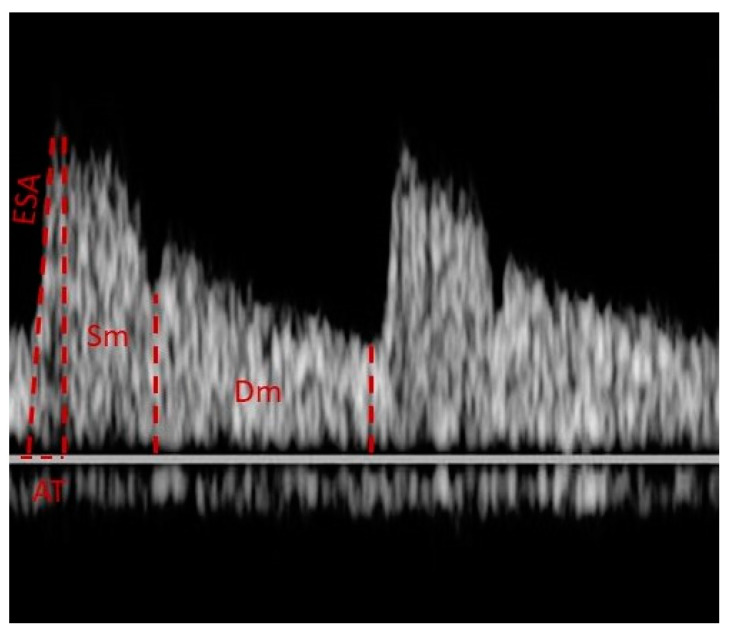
The Doppler waveform of OA. “ESA” corresponds to the inclination of the fastest-moving part of the systolic phase and “AT” to the duration of the above inclination. The “Ratio Sm/Dm” represents the ratio of the mean velocities of the systolic and diastolic phases.
